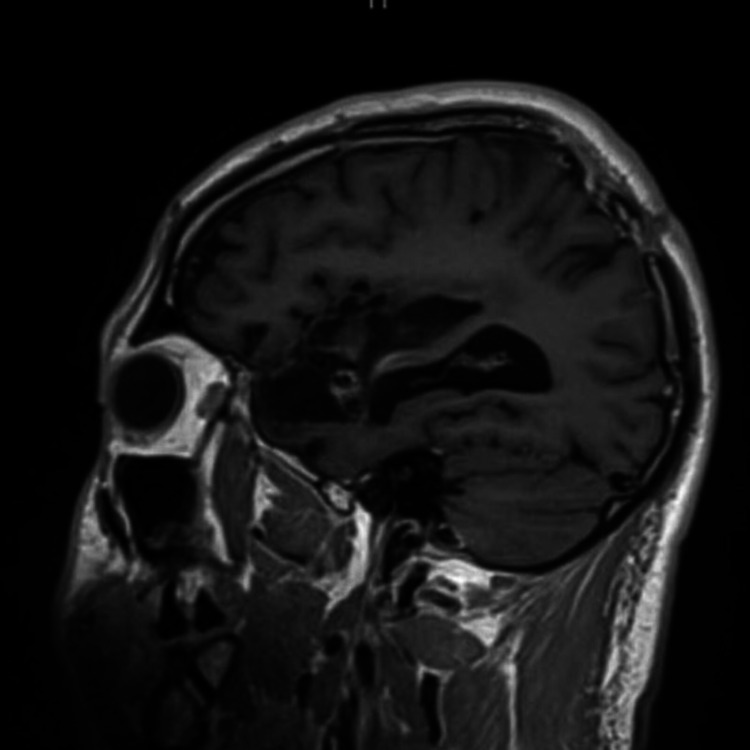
Right frontal arteriovenous malformation (case 1) T1 weighted sagittal section of magnetic resonance imaging, demonstrating arteriovenous malformation in the right frontal area, superior and posterior to the encephalomalacia.
